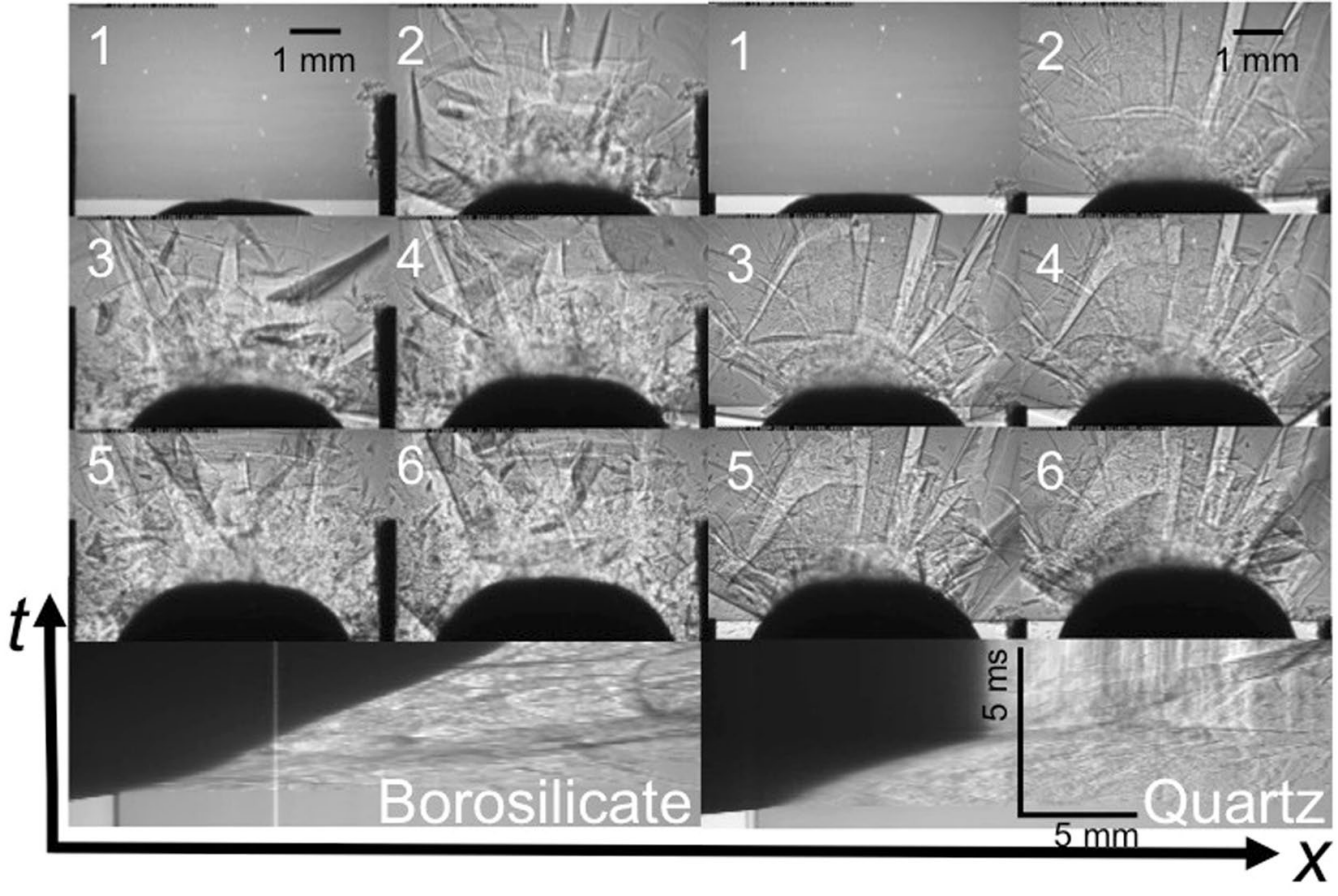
Impact of a spherical indenter. BS radiographic sequence to right and that for quartz to left. The upper part of the figure shows frames from radiographic sequences whilst below each sequence is a streak image for each impact with dark regions showing the indenter and grey-scale reflecting the density of damaged regions down the central axis of the target. Interframe time of *ca*. 68 µs.
